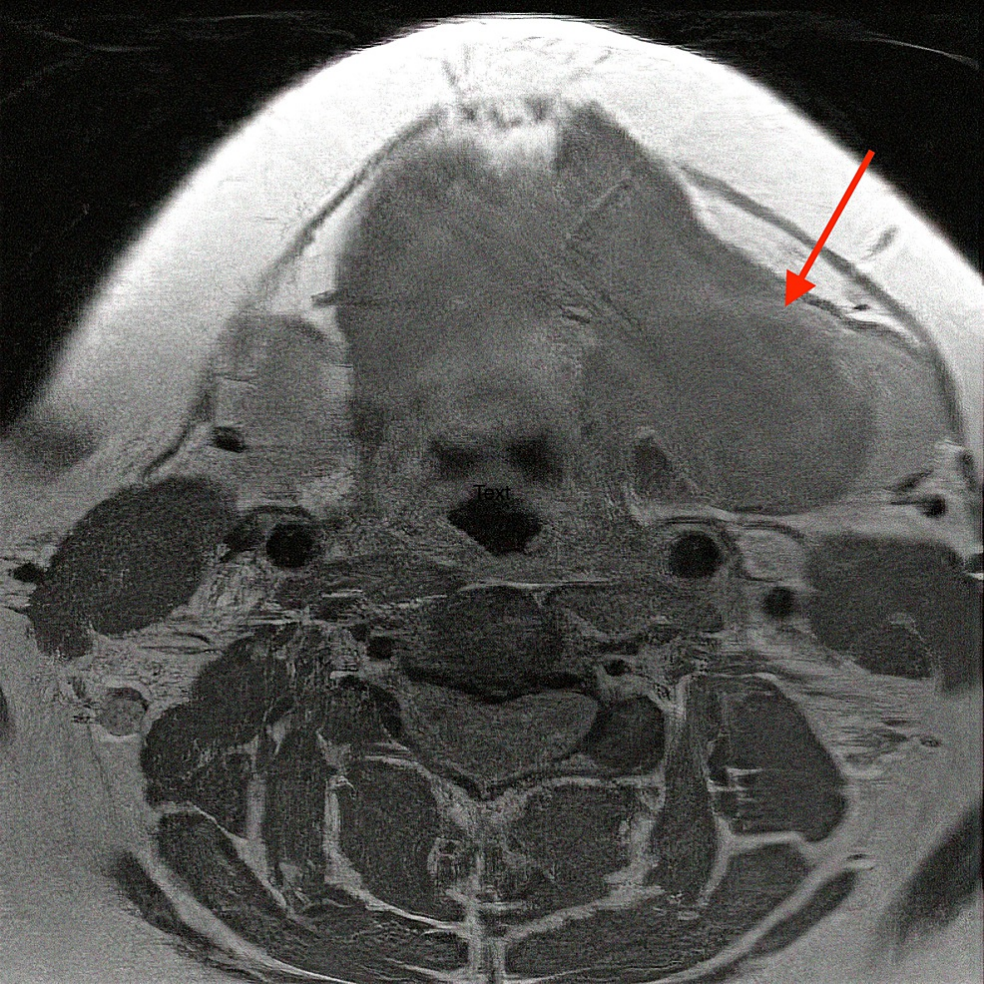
T1-weighted MRI of the head and neck with contrast, axial cut, demonstrating a thin-walled, nonenhancing, elongated cystic mass (red arrow) measuring 4.8 cm x 2.6 cm on the medial aspect of the left submandibular gland.
